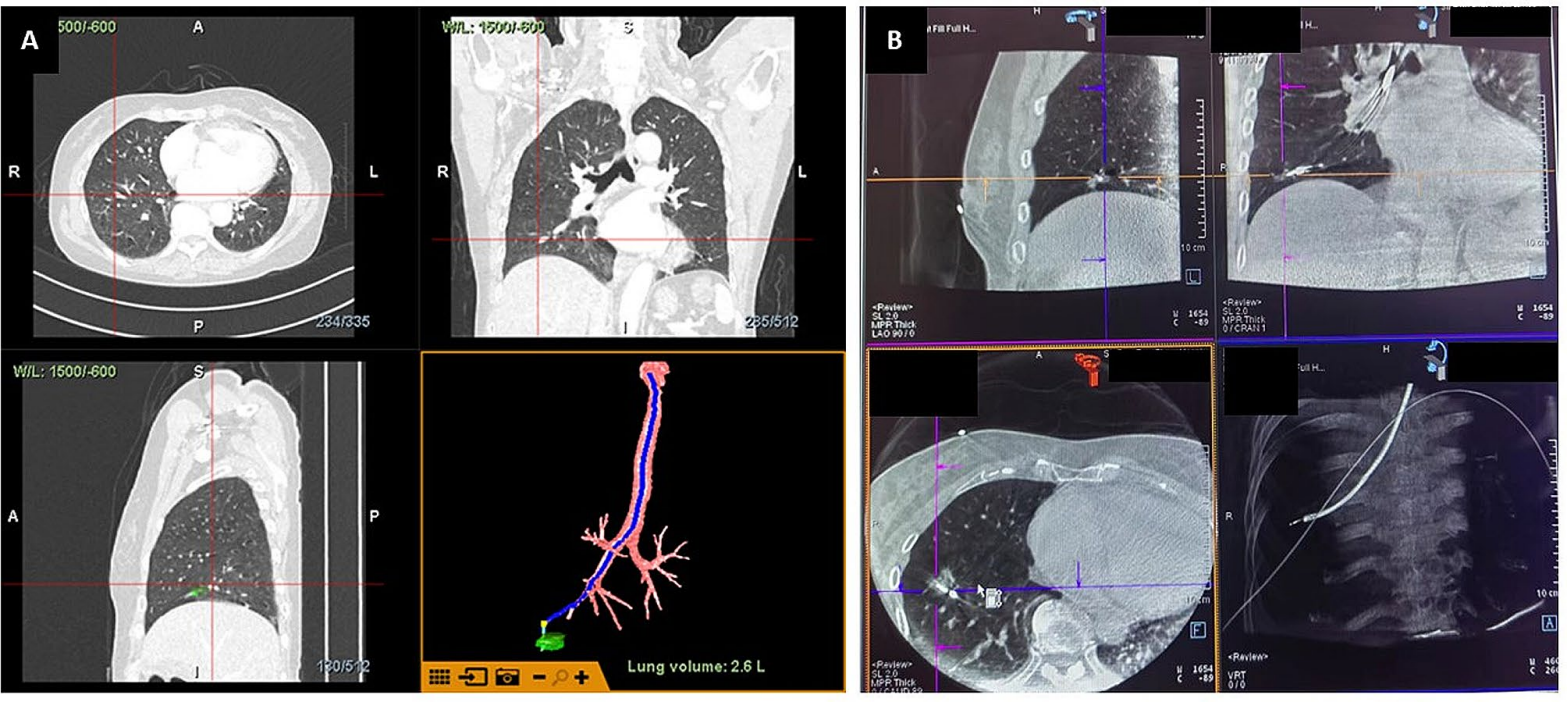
(A) VBN showing the planned path reaching the target lesion; (B) CBCT images from 3 dimensions (vertical, frontal, and sagittal) view showing tip of device within the target. VBN: virtual bronchoscopy navigation; CBCT: cone beam computed tomography
